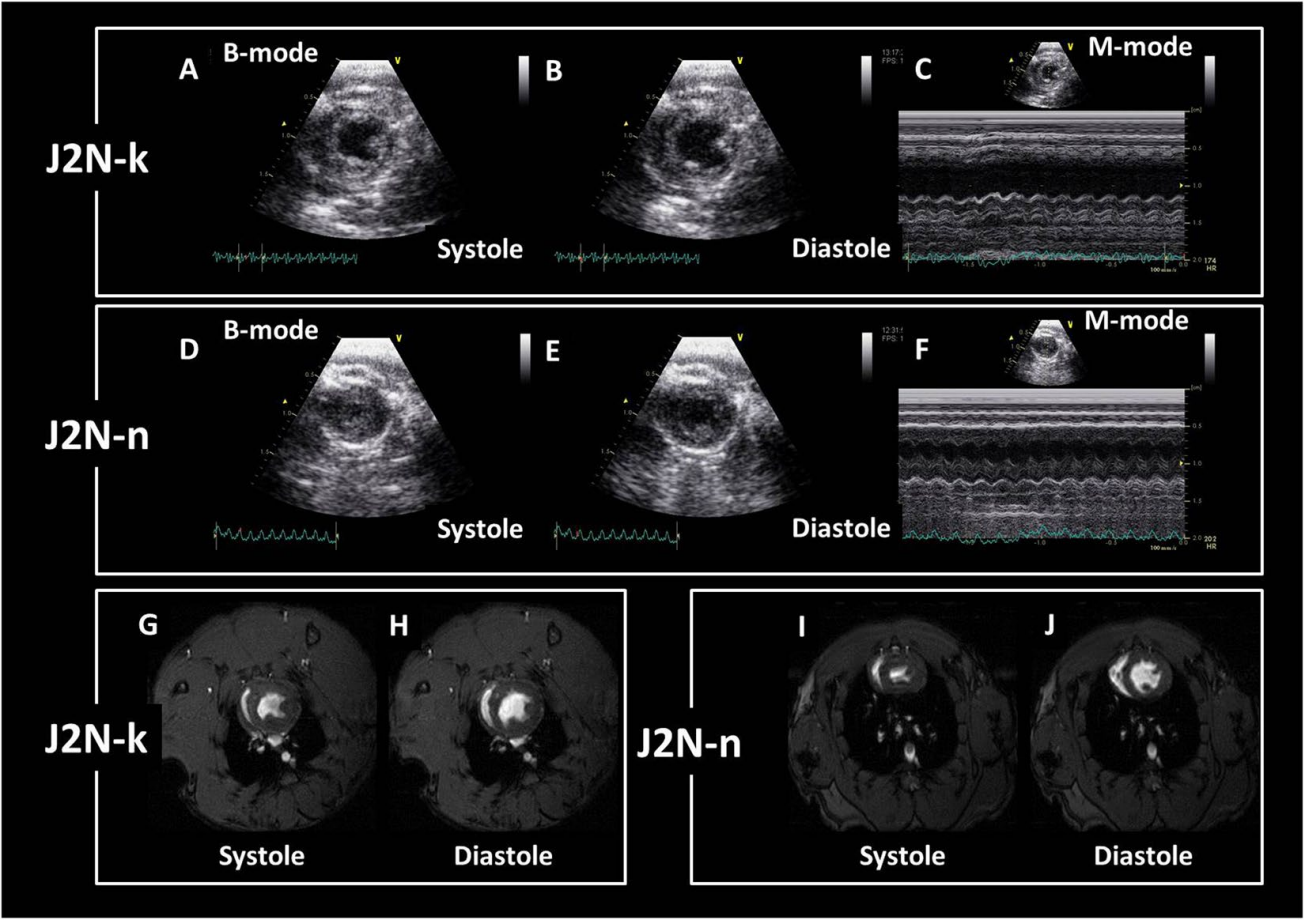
Examples of typical short-axis (SA) view images obtained using echocardiography and magnetic resonance imaging (MRI) for J2N-k cardiomyopathic and J2N-n control hamsters. (A) B-mode SA view images for J2N-k hamsters at end-systole. (B) B-mode SA view images for J2N-k hamsters at end-diastole. (C) M-mode SA view images for J2N-k hamsters. (D) B-mode SA view images for J2N-n hamsters at end-systole. (E) B-mode SA view images for J2N-n hamsters at end-diastole. (F) M-mode SA view images for J2N-n hamsters (G) SA view magnetic resonance images for J2N-k hamsters at end-systole. (H) SA view MR images for J2N-k hamsters at end-diastole. (I) SA view MR images for J2N-n hamsters at end-systole. (J) SA view MR images for J2N-n hamsters at end-diastole.
